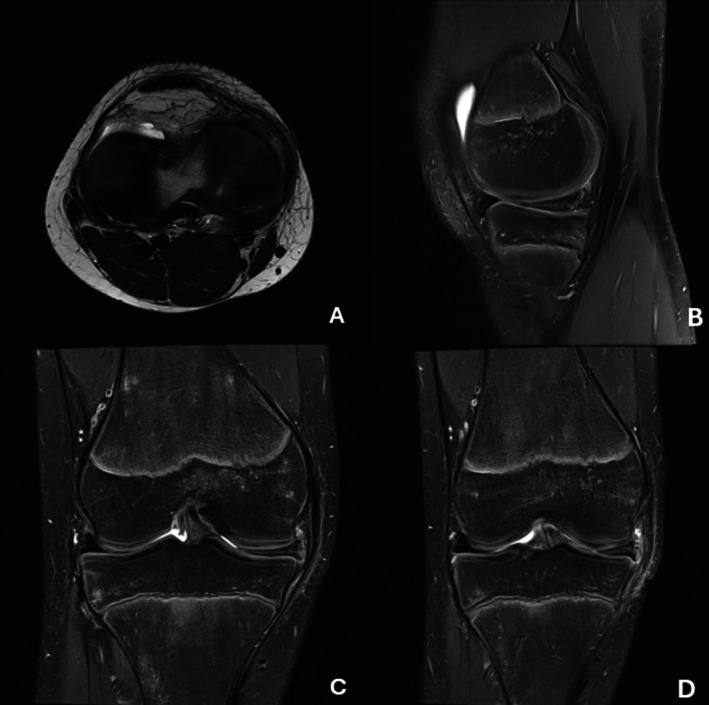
MRI of the right knee. The axial T2‐weighted scan shows a vertical tear at the base of the posterior horn (A). The sagittal T2 scan shows a high signal intensity in the medial meniscus, indicative of a vertical tear (B). Coronal view shows a horizontal cleavage tear in the medial meniscus (C, D).
